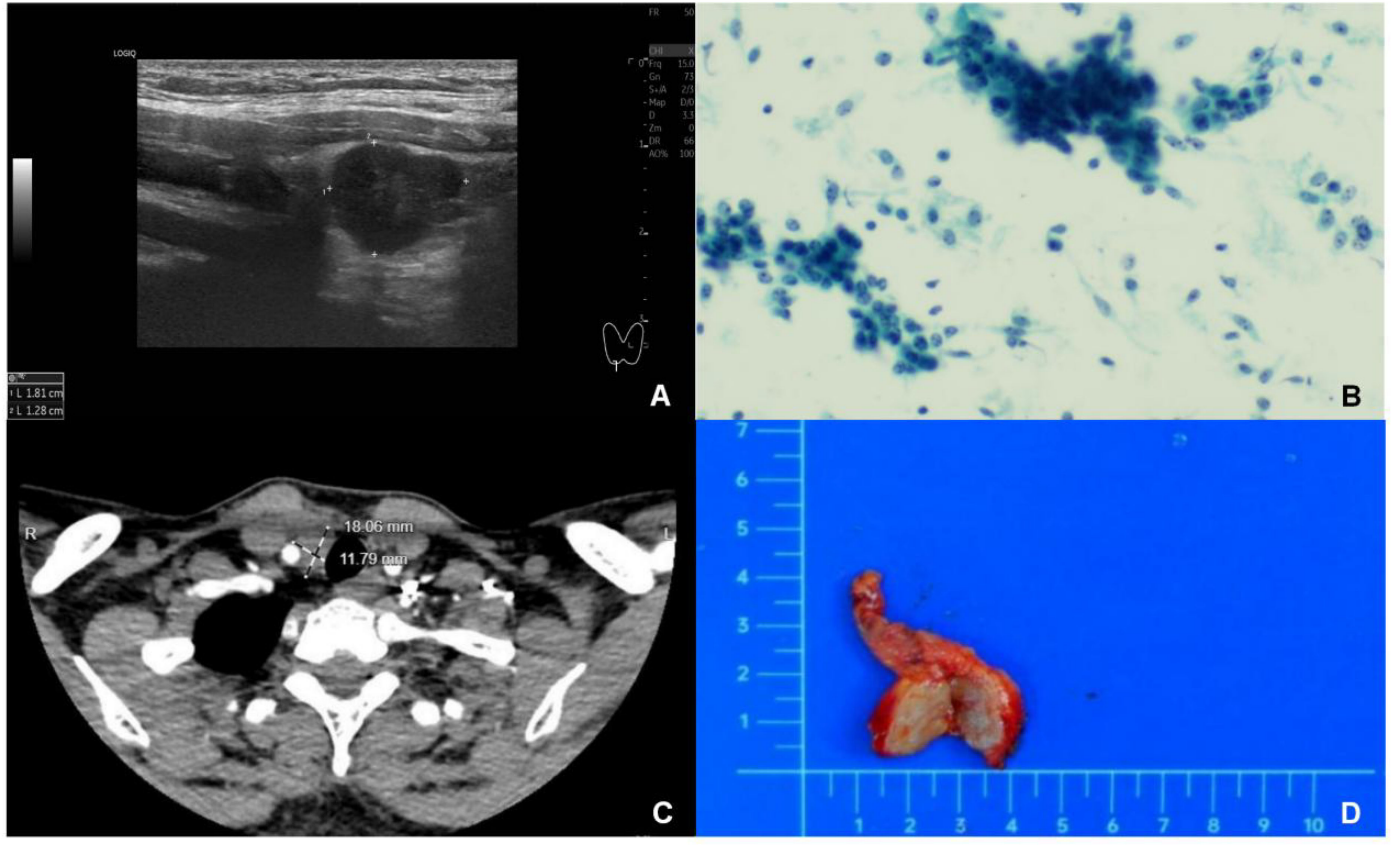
Intrathyroidal thymic carcinoma. (A): Ultrasonography revealed a solid mass measuring 18×13 mm located beneath the right thyroid gland. The mass exhibited hypoechogenicity, with well - defined margins and a regular shape. (B) Fine-needle aspiration biopsy showed cells arranged in clusters, sheets, and scattered. They were crowded and disorganized, with round, oval, or short - spindle shapes. Most cells were similar, but a few had enlarged, atypical nuclei with 1–3 distinct nucleoli and nuclear displacement. (C) CT scans showed the mass was hypodense with clear margins. It had mild to moderate enhancement after contrast injection, no obvious calcification, and was adjacent to the right common carotid artery and trachea. (D) Gross examination revealed a solid tumor with clear margins, regular shape, grayish - yellow cut surface, and no obvious capsule.
